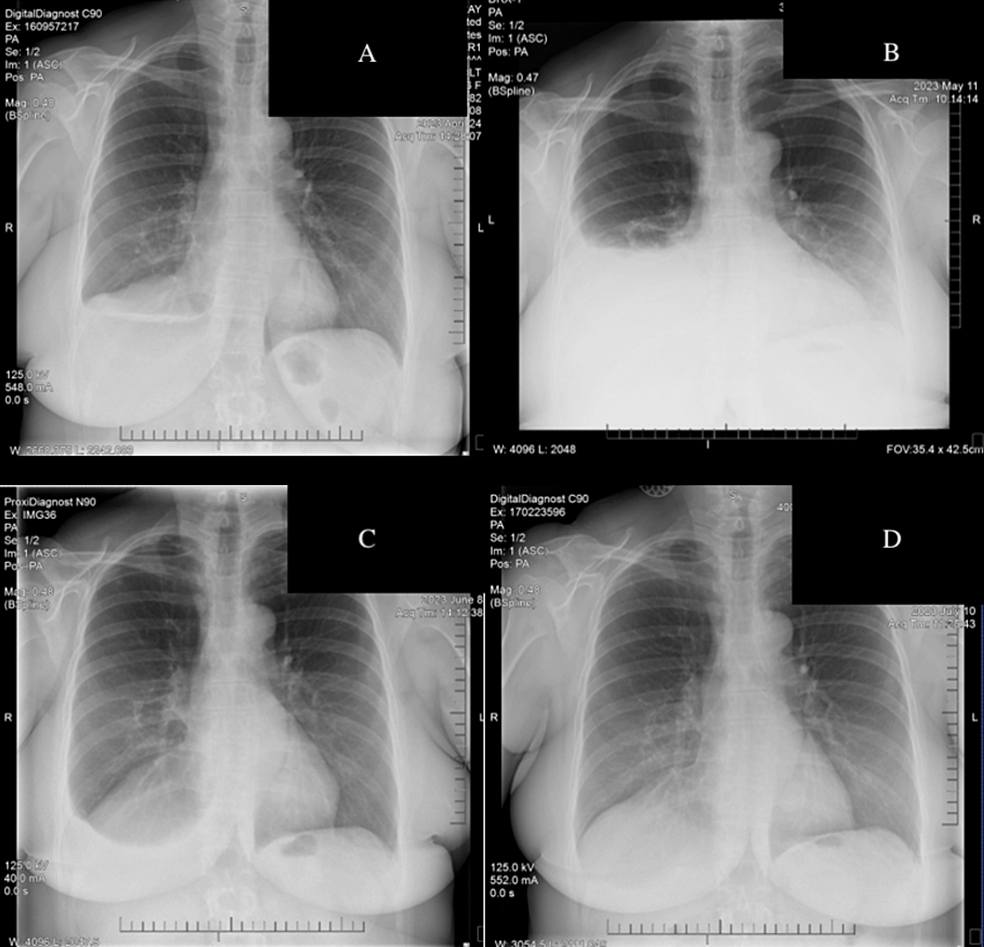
Chest X-rays showing right-sided pleural effusion resolution (A) After last thoracentesis, (B) four weeks after changing diet and two weeks after last thoracentesis, (C) seven weeks after changing diet and five weeks after last thoracentesis, (D) eleven weeks after changing diet and nine weeks after last thoracentesis.
